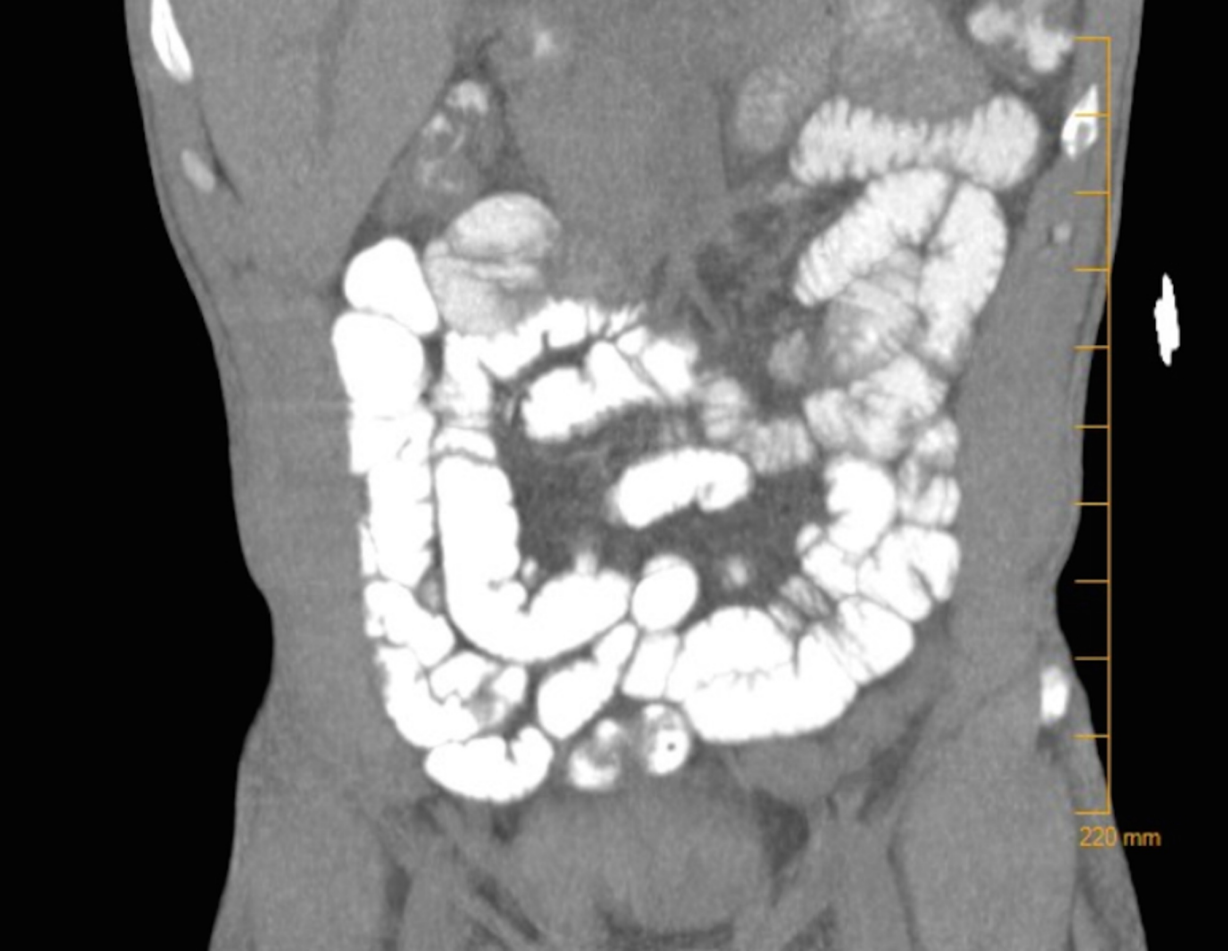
A computed tomography scan showing diffuse bowel wall thickening from the terminal ileum throughout the entire colon
